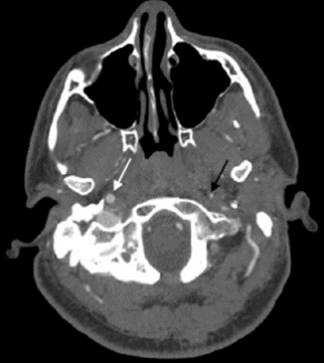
Axial computed tomography demonstrating significant stenosis of the left internal carotid artery (black arrow) vs the patent right internal carotid artery (white arrow).
